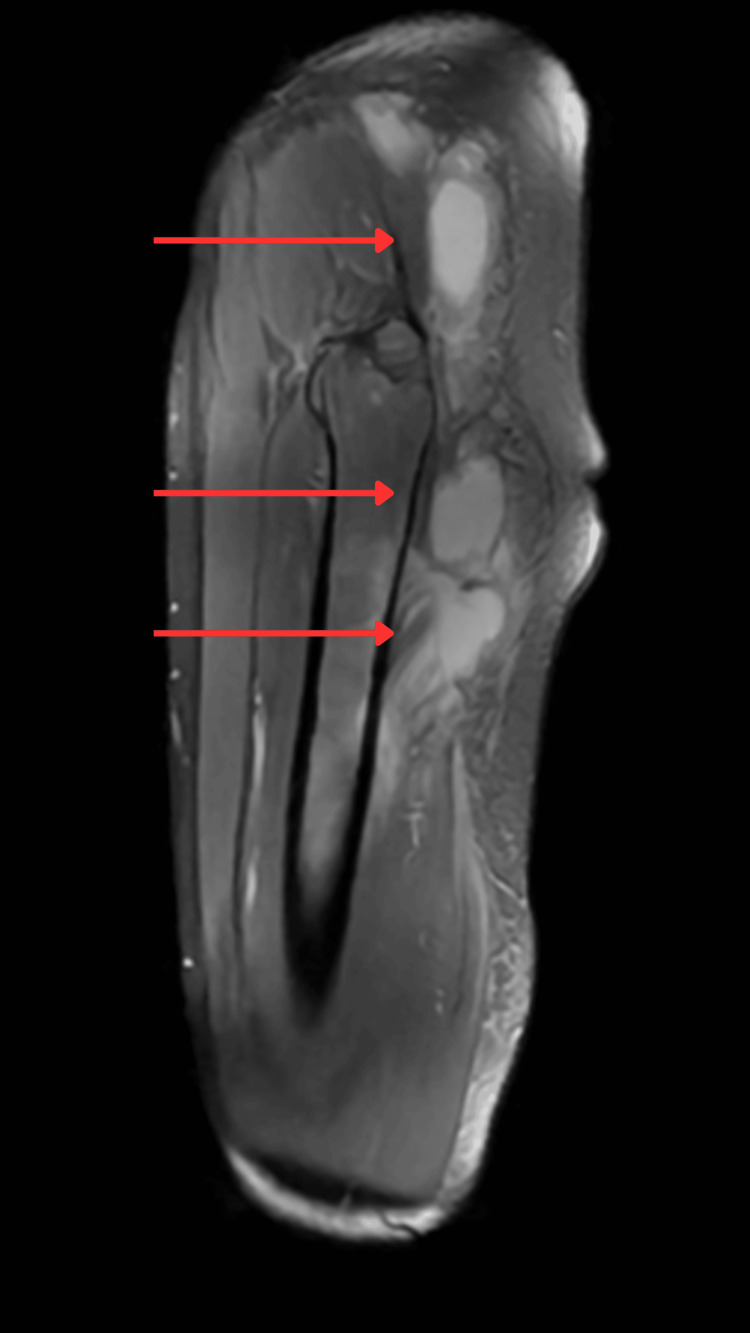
MRI left thigh showing a multilobulated abscess indicated with red arrows.
